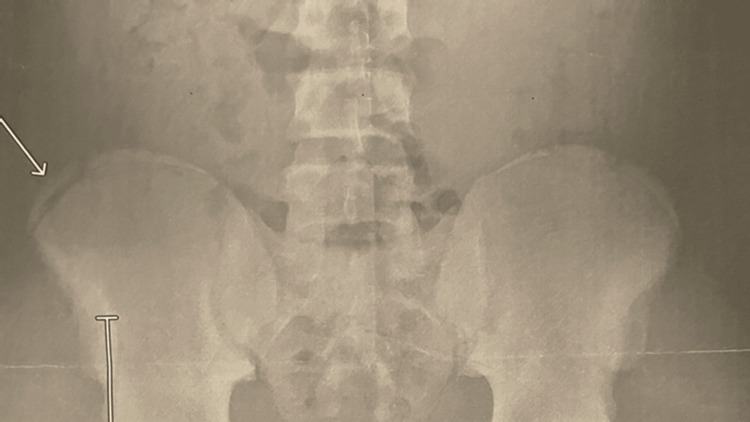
Radiograph of the right iliac crest The arrow denotes the apophyseal injury.
